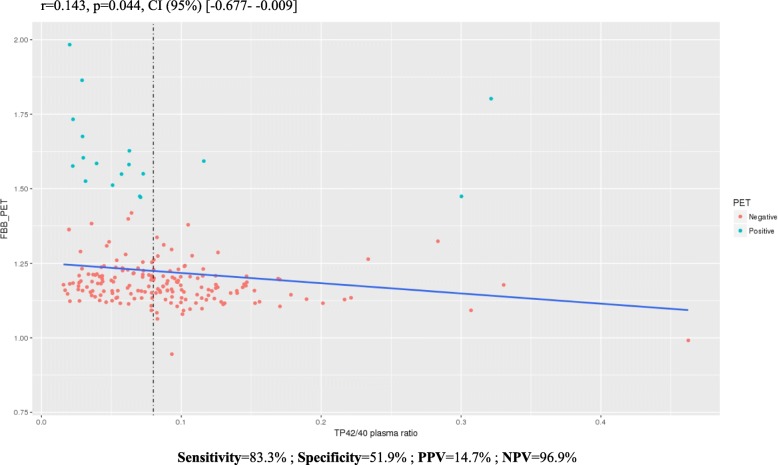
Linear regression between florbetaben(^18^F) (FBB)-positron emission tomography (PET) global SUVR and Aβ total plasma (TP)42/40 plasma ratio in SCD subjects. Inverse association between Aβ TP42/40 plasma ratio and FBB-PET scan. Experimental cut-off point of Aβ plasma ratio TP42/40 established at 0.08 to reduce the prescreening number of Aβ FBB-PET scans to 52.8%. CI confidence interval, NPV negative predictive value, PPV positive predictive value
